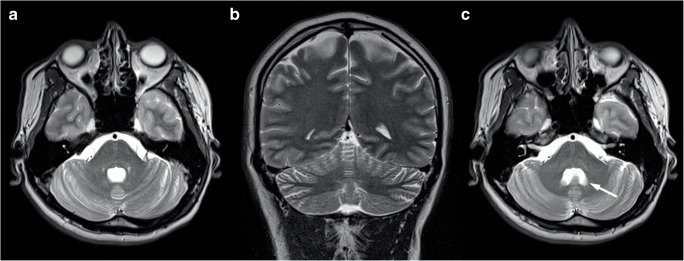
Axial (a, c) and coronal (b) T2-weighted images showing mild cerebellar atrophy, with enlargement of the 4th ventricle (arrow), in a 30-year-old female SCA3 patient
